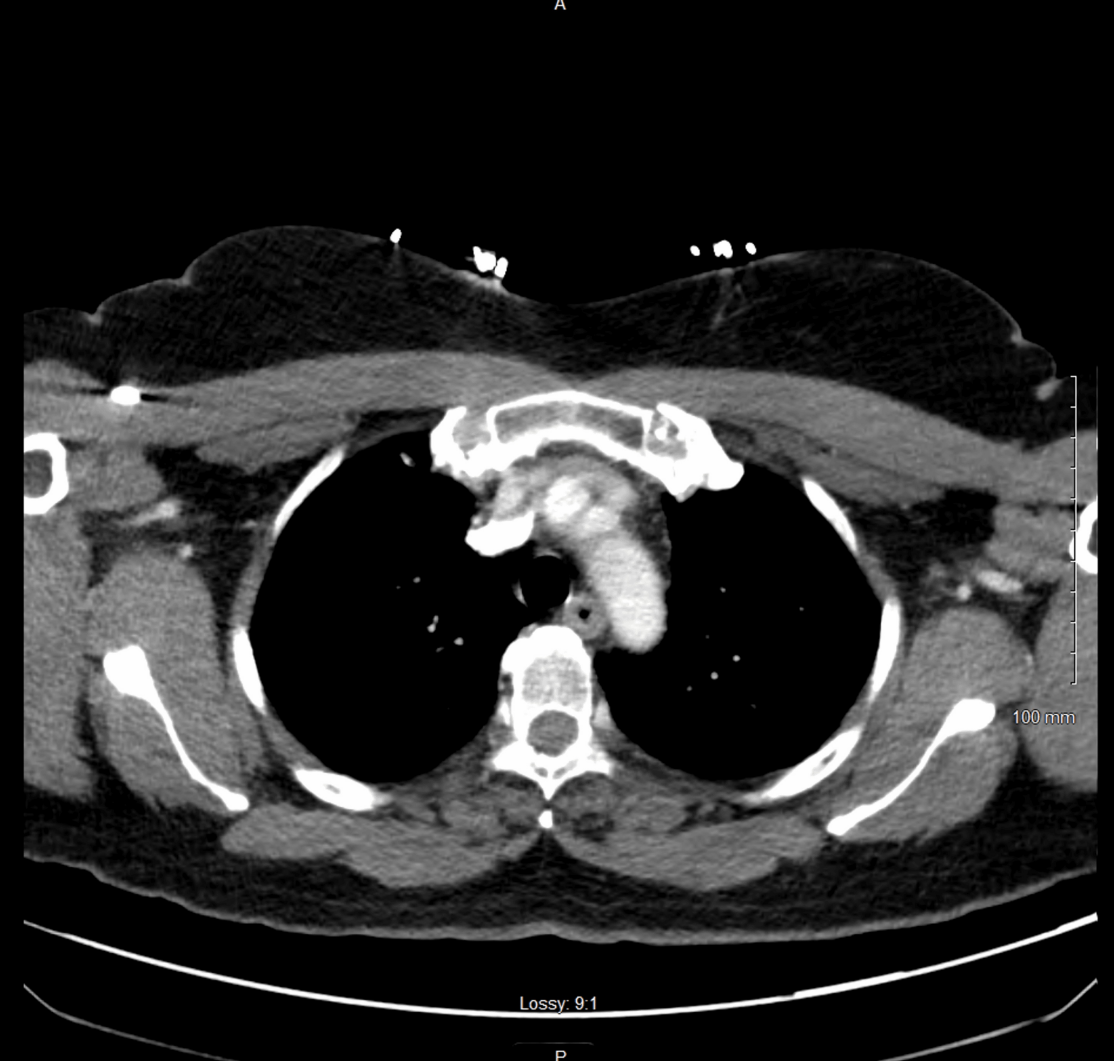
Computed Tomography of Abdomen and Pelvis
